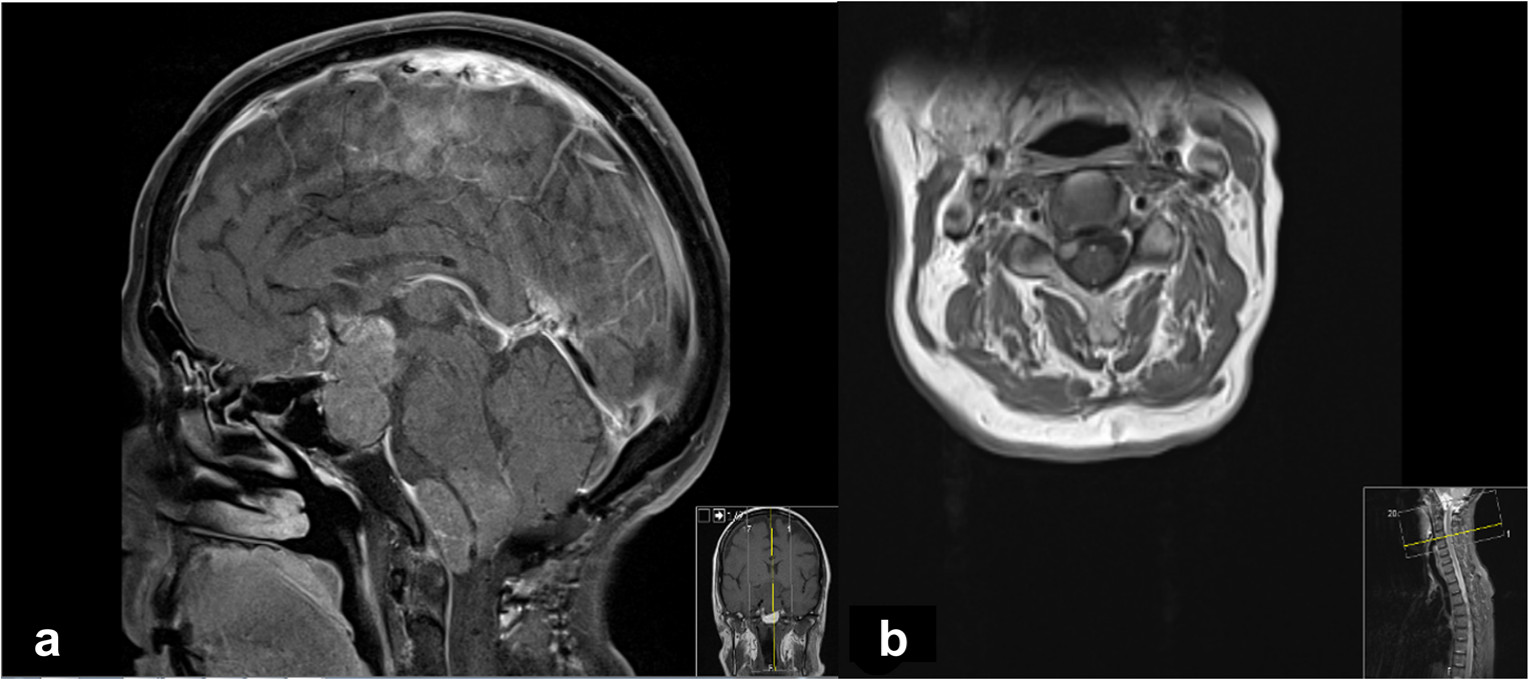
Radiological images of the pituitary lesion and metastatic deposits. a Sagittal section of MRI pituitary post gadolinium showing suprasellar mass and extra-axial metastatic deposit in the posterior fossa with extension into the left hypoglossal canal. b MRI axial sequence post gadolinium showing the intradural extra-medullary cervical metastatic deposit at the level C3/C4
